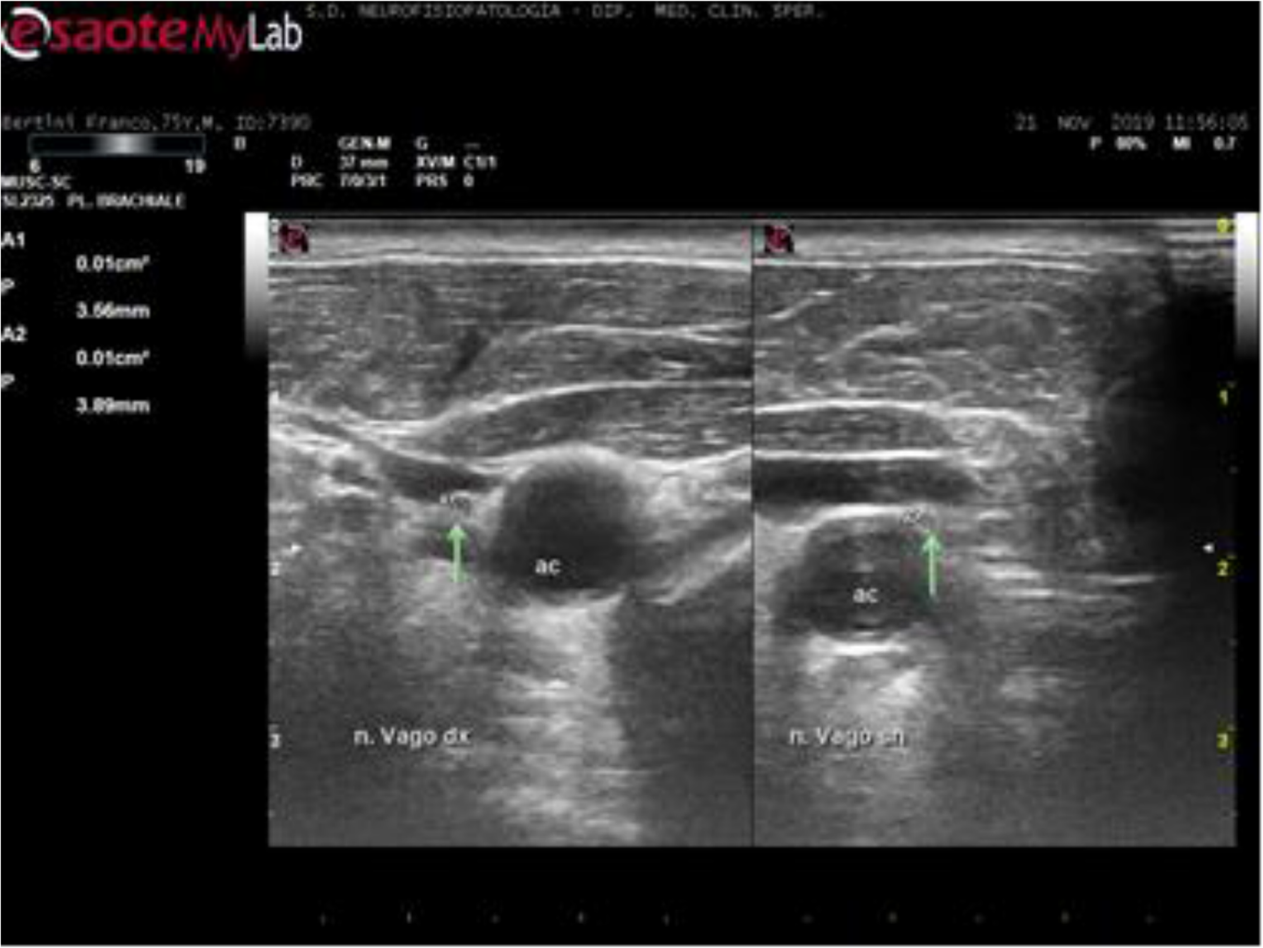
Typical high-resolution ultrasonographic findings of VN on both sides (arrow) in a patients with Parkinson’s disease (case BF, M, 75 years old, disease duration 9 years, hyperkinetic–hypertonic type). Note the substantially symmetric clearly reduced CSA of VN and the presence of a thin hyperechoic rim and no appreciable internal structure. ac, carotid artery
